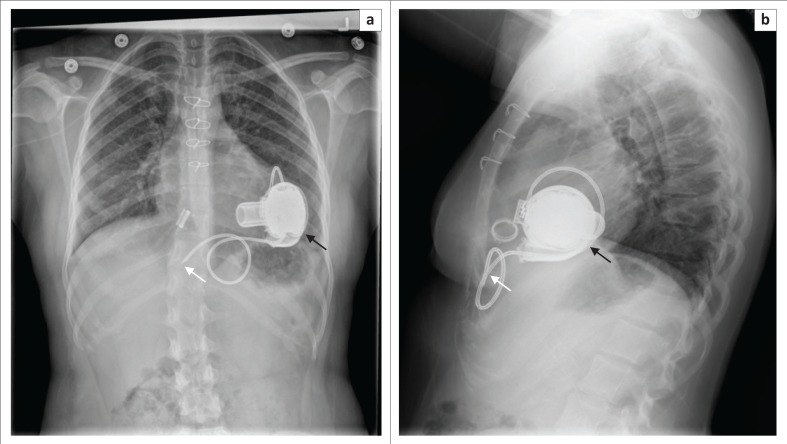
Frontal (a) and lateral (b) chest radiographs showing a decommissioned HeartWare left ventricular assist device (thick arrow) with an intentionally severed driveline (arrow) in a 33-year-old lady with a history of left coronary dissection.
